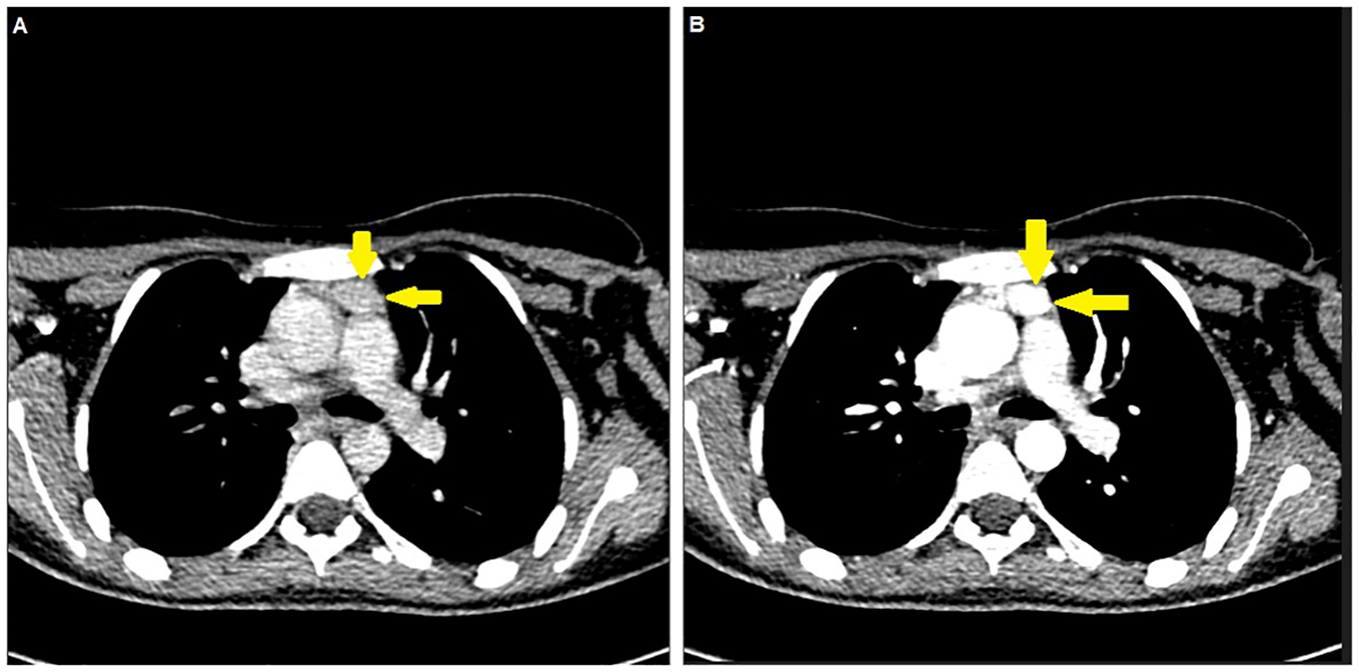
An ectopic thymic parathyroid adenoma detected by (A) neck (venous phase) computed tomography and (B) thoracic (arterial phase) computed tomography (yellow arrows).
